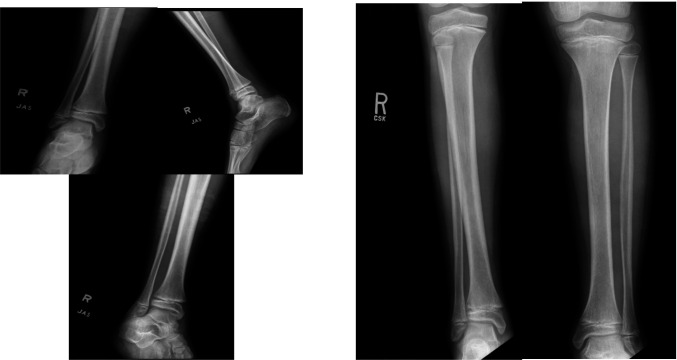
Initial radiographs of the patient's distal tibia. A Salter-Harris II fracture can be seen on the lateral radiograph. No obvious rotational deformity is appreciable on these images. The ankle mortise appears intact. The AP full-length radiographs of the right and left tibia demonstrate asymmetry.
